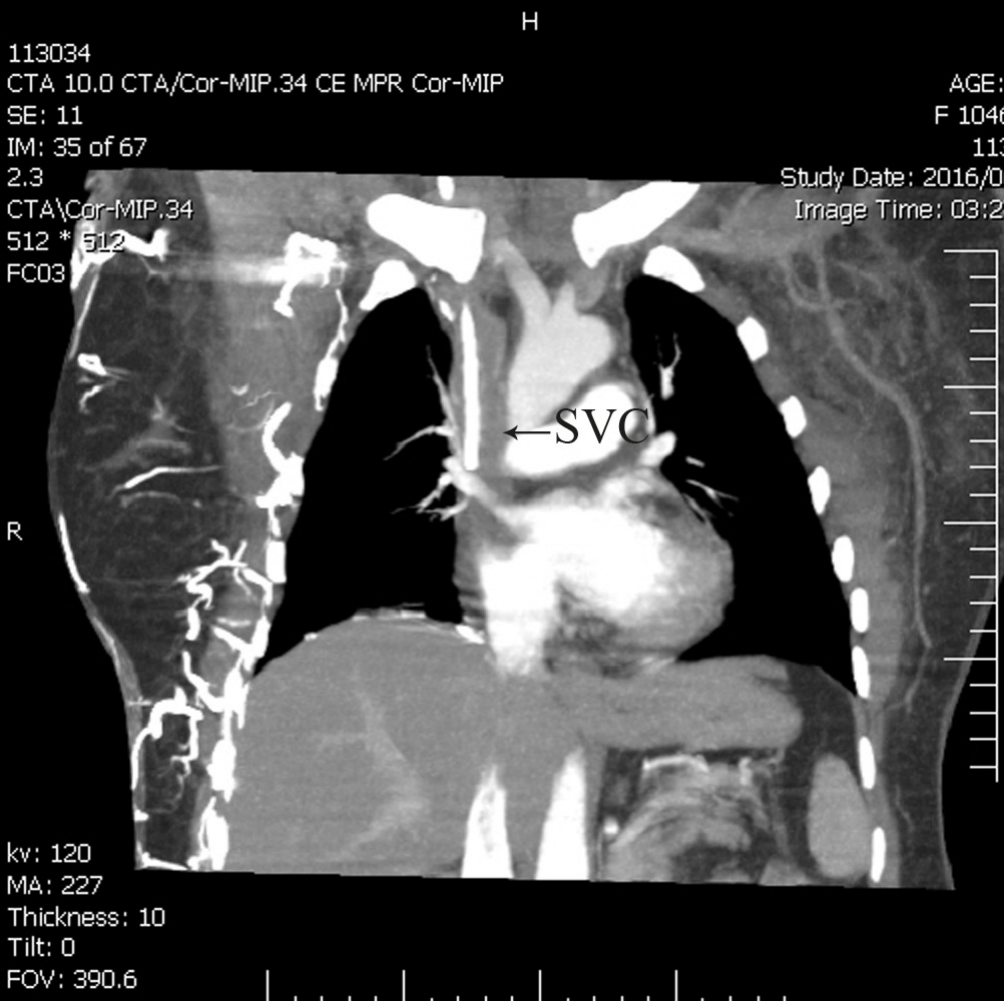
Computed tomography scan with contrast in the posterior anterior projection, prior to catheter-directed thrombolysis, showing an obstructed SVC (arrow) with many enlarged collaterals.
